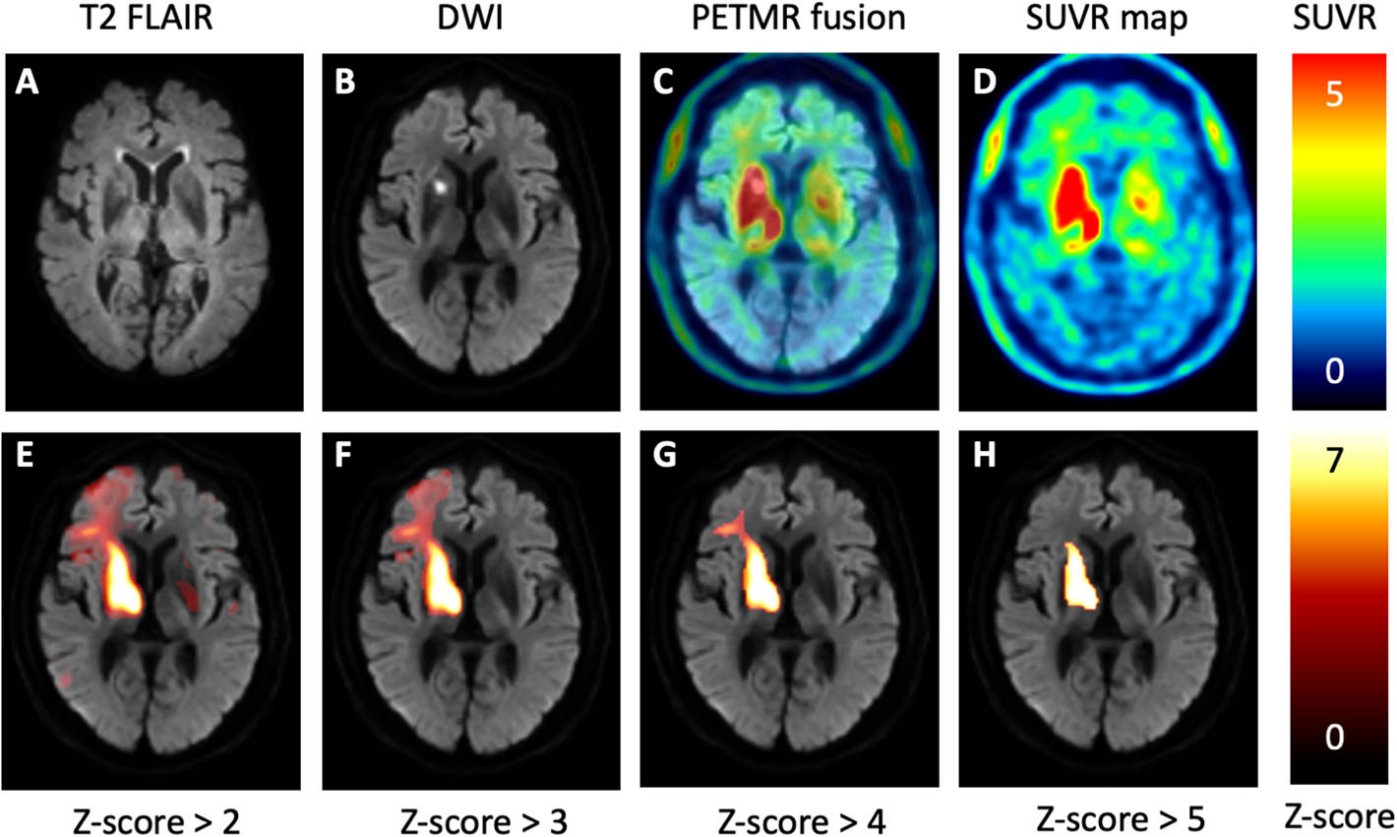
Comparisons of ^18^F-THK-5351 uptake patterns among the SUVR map and Z-maps at multiple Z-score thresholds. A patient with a lacunar infarct at the right internal capsule (a, b) shows increased ^18^F-THK-5351 uptake around the stroke lesion on the SUVR maps (c, d). On the Z-maps with sequentially increasing thresholds, the ^18^F-THK-5351 uptake regions gradually shrink (e–h). Furthermore, the increased ^18^F-THK-5351 uptake might extend to the cerebral cortex without corresponding changes observed on the conventional FLAIR and DWI images
